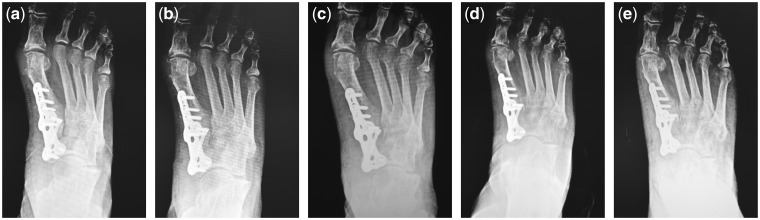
Follow-ups of clinical outcomes by X-ray examinations. Transverse section X-ray examinations at the 2nd, 4th, 6th, 9th and 13th weeks after surgical operation were displayed from a to e, respectively. The metatarsal-cuneiform joint and navicular-cuneiform joint of right lower extremity was perfectly stabilized with an internal fixation plate and screws. On part e, we can see complete consolidation of the medial column with excellent coalescence at the medial column without any lucency visible at the fusion sites. This is one-and-half times faster than expected for this type of surgery
